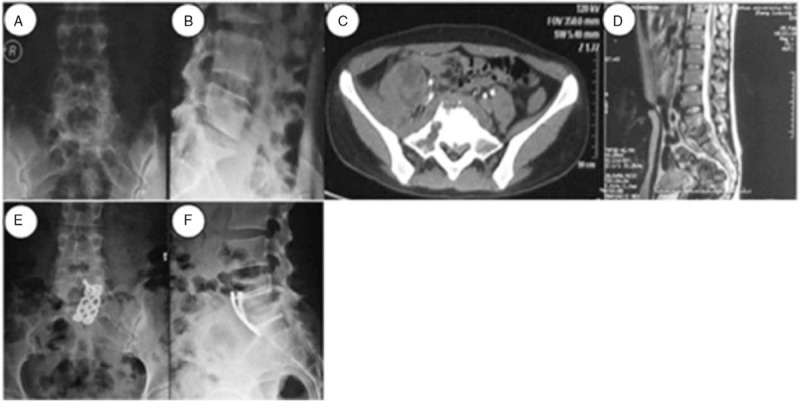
A 26-year-old woman was diagnosed with L5-S1TB and underwent anterior debridement and reconstruction with anatomical screw-plate fixation. Before surgery, posteroanterior (A) and lateral (B) plain radiographs showed the L5–S1 vertebral damage with a narrowed intervertebral space and a decreased lumbosacral angle. The CT (C) and MRI (D) scans showed the destruction of L5 and S1 vertebrae, with a cold abscess compressing the neural elements. During the last follow-up visit, the x-ray images (E and F) showed a complete correction of the lumbosacral angle and a solid fusion.
